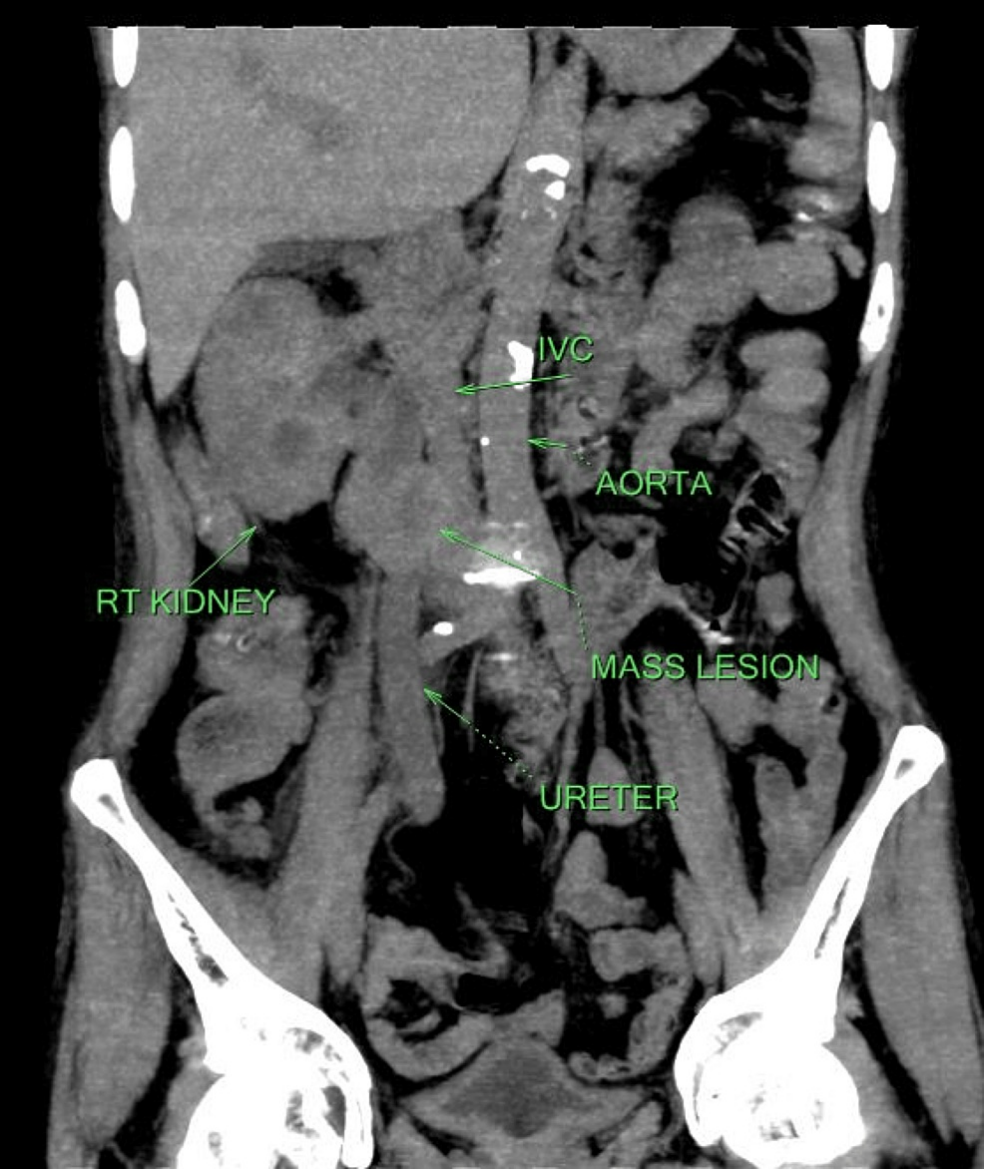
Non-contrast computed tomography scan showing mass lesion along with right ureter and inferior vena cava
